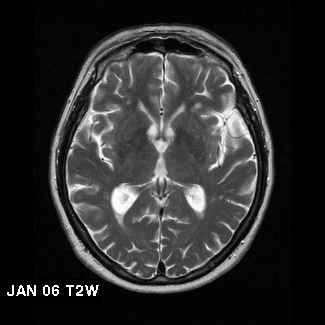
Follow-up radiological findings in Case 7: T2 weighted MRI scans of Case 7 showing extent of white matter disease in January 2006
